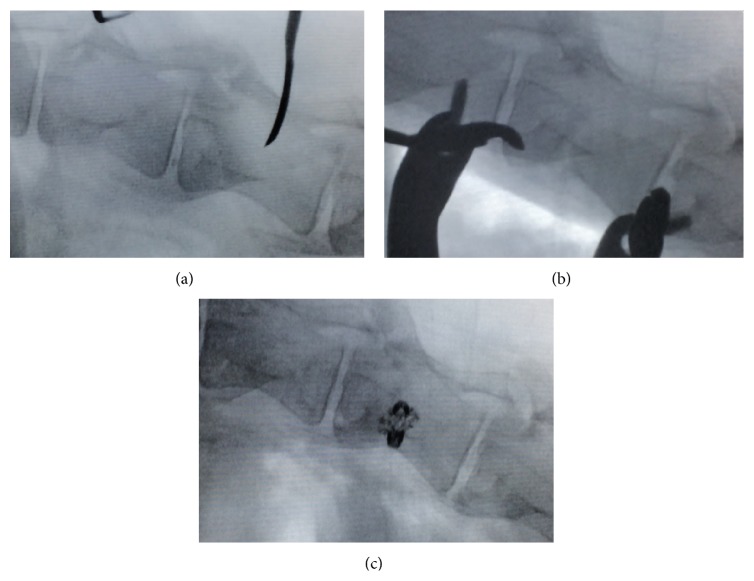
Intraoperative radiographic imaging preoperative (a), after fracture creation (b), and postinterventional VCF fixation by extrapedicular placement of the titanium mesh cage (c).
